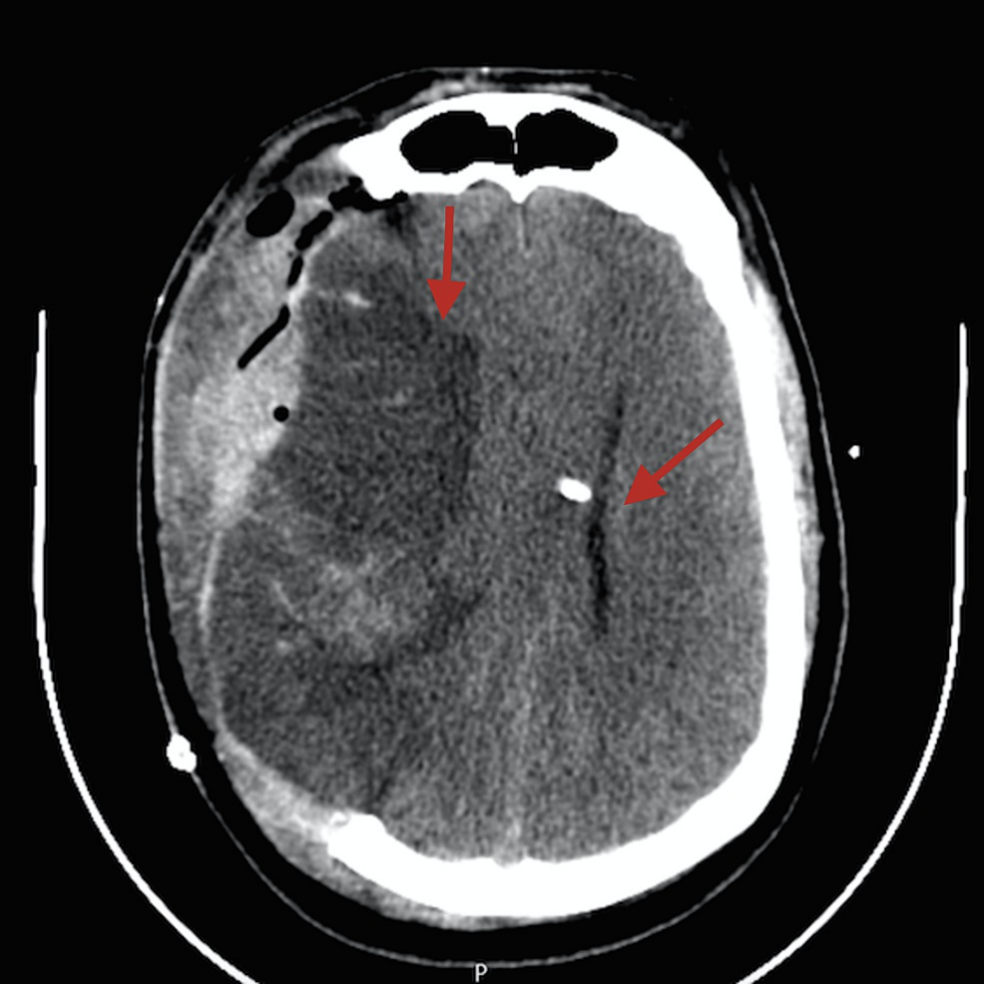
Cerebral CT scan showing ischemic infarction in the territory of the right middle cerebral artery with hemorrhagic transformation, leading to worsening of the mass effect, effacement of the perimesencephalic base cisterns, compression of the ventricular system, and deviation of the midline structures by 11 millimeters.
